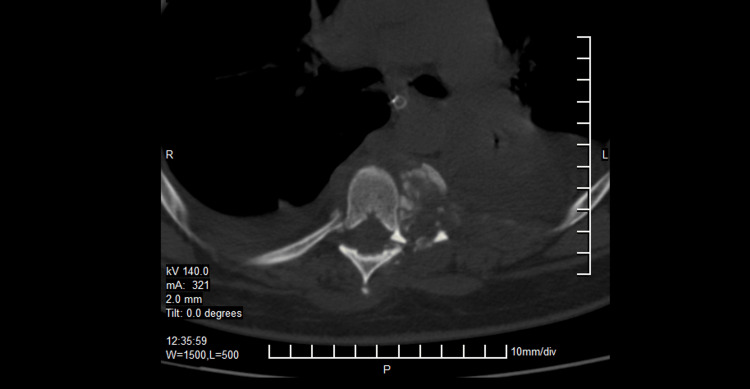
Case 1 CT scan axial cut showing the lateral displacement of the bodies and the loss of the relationship between the vertebral body and the spinal canal.
